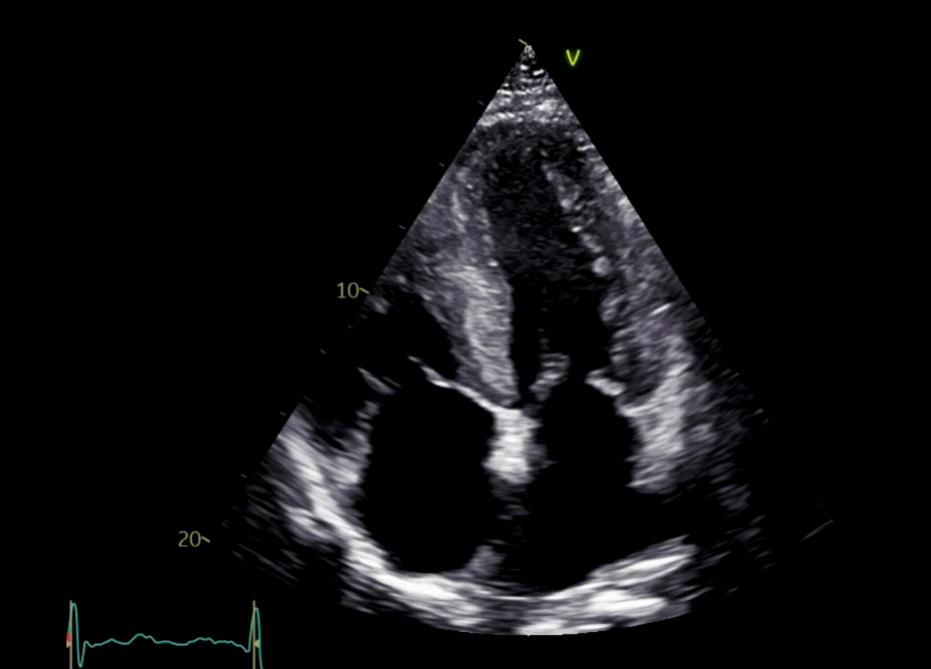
Family 1, Patient 1: transthoracic echocardiography in a four-chamber view, showing severe wall thickening and atrial dilatation.
